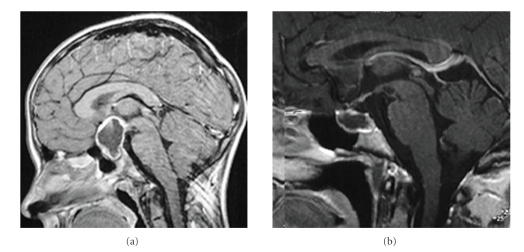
Obesity and daytime sleepiness in relation to the localization of craniopharyngioma. The patient whose preoperative MRI (a) showed a large tumor extending to the suprasellar region and infiltrating the hypothalamus developed severe daytime sleepiness and, consequently, obesity (BMI: +14 SD [4]). The patient with a childhood craniopharyngioma of intrasellar localization seen in Figure 1(b) maintained normal weight (BMI: +1 SD [4]) and developed no daytime sleepiness.
